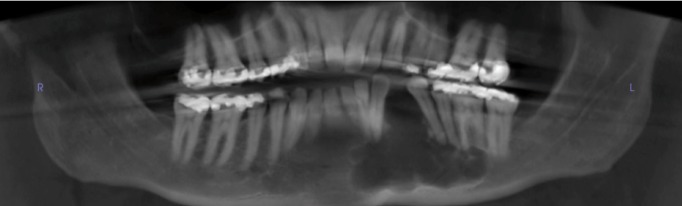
Panoramic radiograph at first presentation. This panoramic radiograph was taken at first presentation and displays a well-demarcated, multilocular radiolucency with scalloped border between teeth #19-23. The teeth are pushed apart, especially area between #21 and 22.
